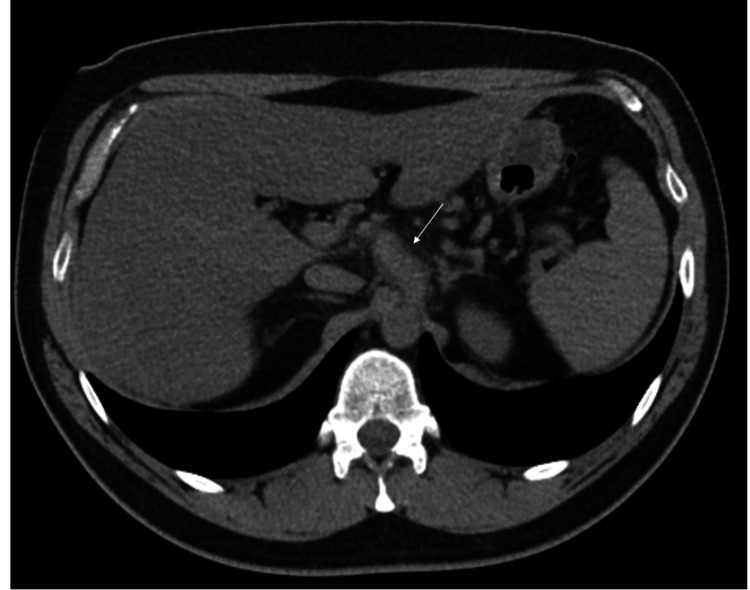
Axial non-contrast CT of the abdomen. Fusiform dilatation of the celiac trunk (white arrow) is evident immediately after its origin from the abdominal aorta. The dilated celiac trunk demonstrates areas of hyperattenuation (+61 HU) and surrounding fat stranding.
